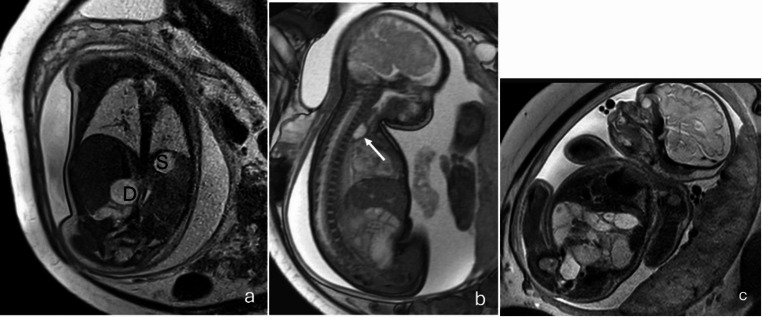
Multiple levels of atresia. In case 1 (a, b), MRI exam was performed at 30GW after US diagnosis of polyhydramnios and duodenal atresia. Coronal T2-W image (a) shows a classical “double-bubble sign” with a distended stomach (S) and duodenum (D). The midline sagittal T2-W image (b) reveals a “cervical pouch sign” (arrow), not previously detected. In case 2, a coronal T2-W MR image (c) at 34 GW shows distended intestinal loops filled with fluids with different signal intensities, indicating multiple levels of obstruction
